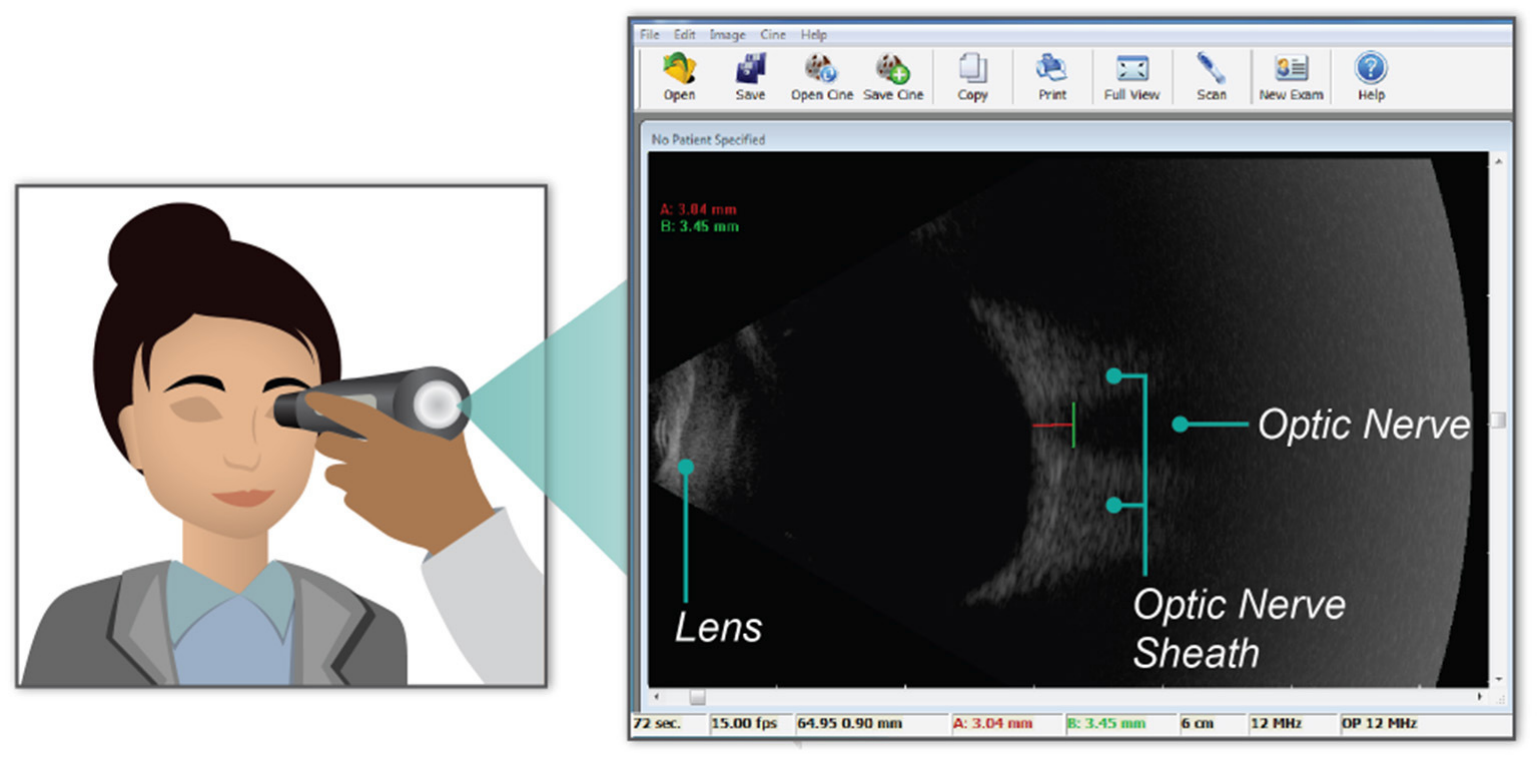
Graphical representation of the placement of the ultrasound probe over the subject's closed eye lid (*left*). The blowout image (*right*) shows a single frame within the video recorded during data collection. Key anatomical landmarks (i.e., the lens, optic nerve, and the optic nerve sheath) are labeled in the ultrasound image. Further, the green line on the ultrasound image shows the data measurement made of the optic nerve sheath diameter. In this example, the diameter is 3.45 mm.
